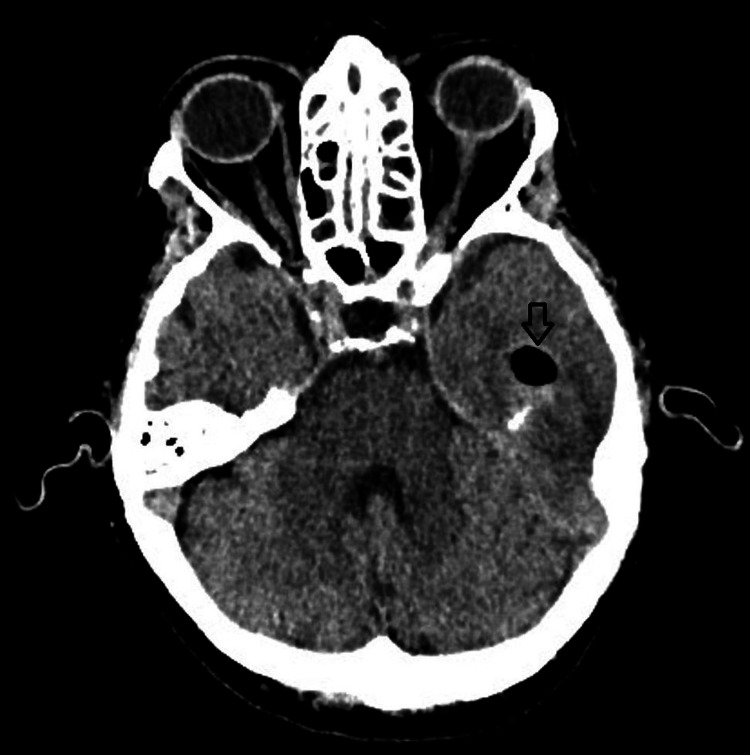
A plain CT scan of the brain showing a 12mm air-filled cyst in the left temporal lobe with mild surrounding white matter edema
